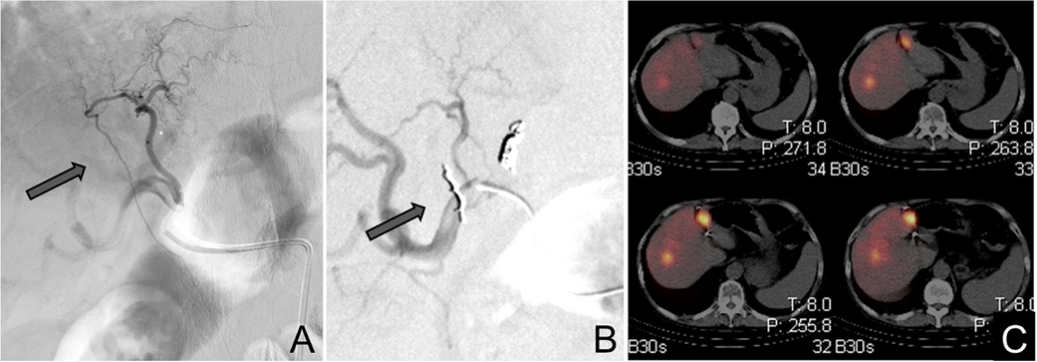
Example of a coilable hepatic falciform artery (HFA). Primarily patent HFA visible in the pretherapeutic DSA (A) which was successfully coil embolized (B). Afterwards no abdominal wall ^99m^Tc-HSAM acculumulation was detected in the SPECT/CT (C).
